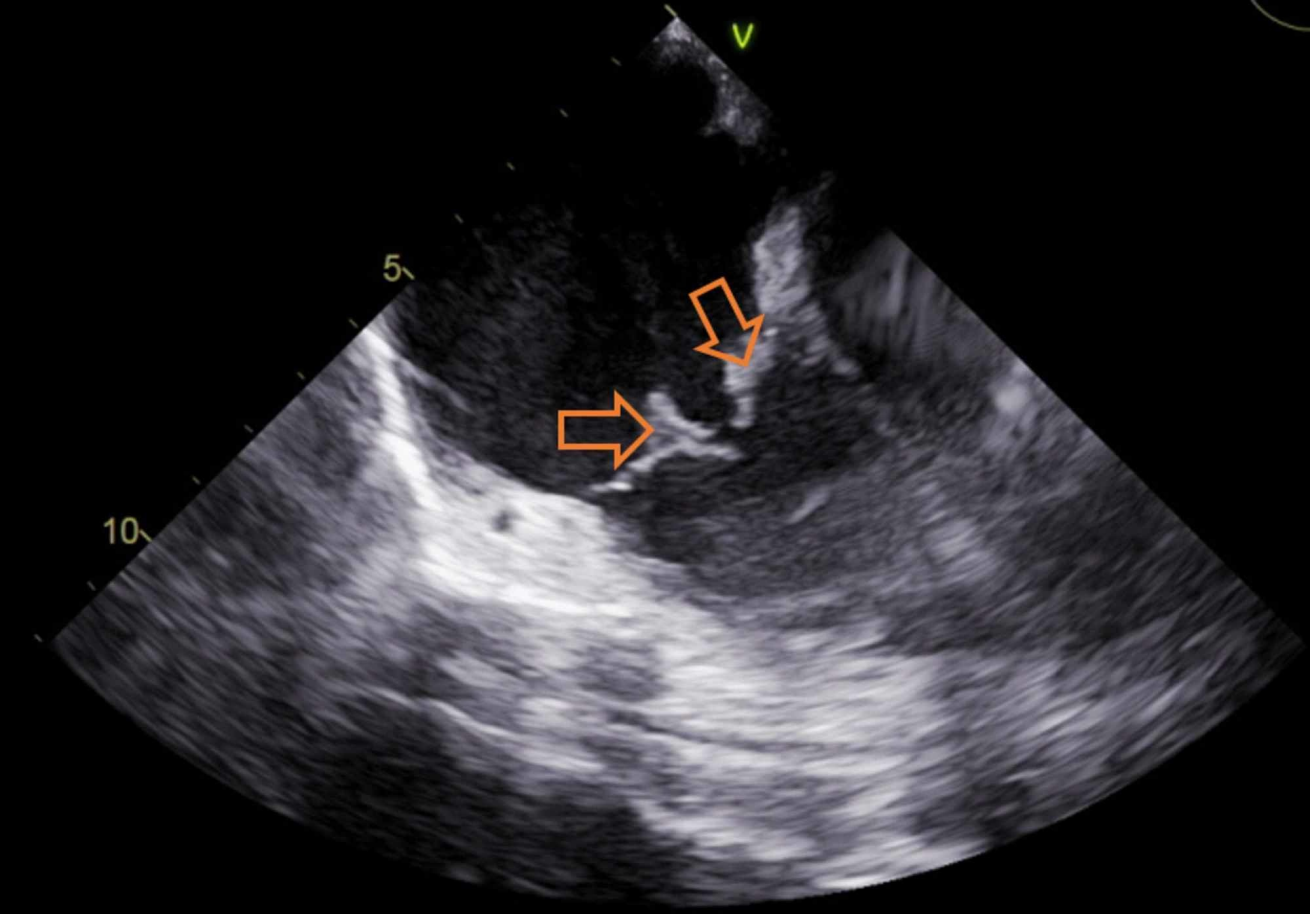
Postprocedure transesophageal echocardiogram (TEE) TEE following percutaneous aspiration of tricuspid vegetations showing significant reduction in bulk of both masses.
